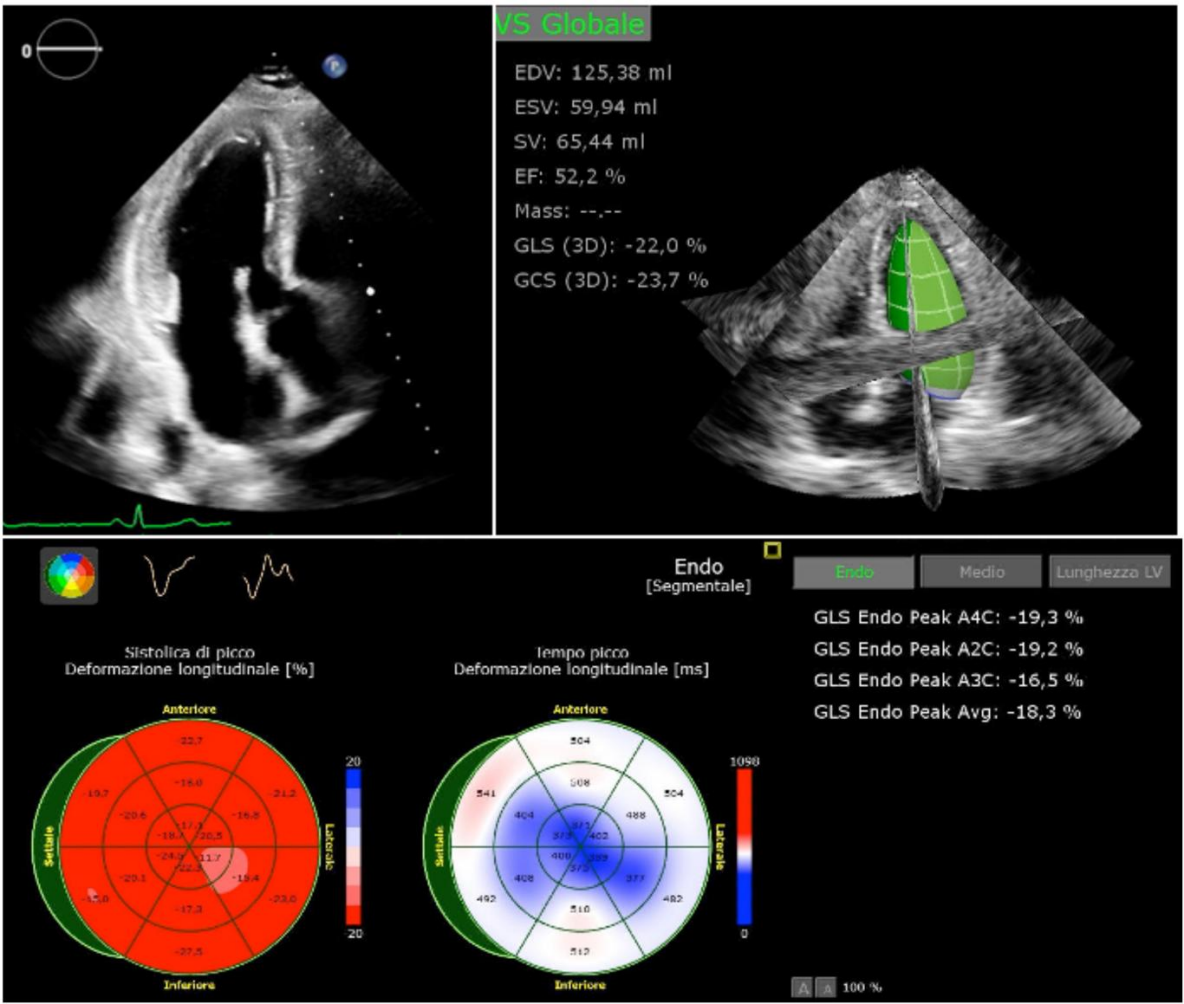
Transthoracic echocardiography. (*Top left panel*) Three-chamber view; (*Top right panel*) 3D echocardiographic ejection fraction and volumes; (*bottom panel*) percentage systolic peak longitudinal deformation index.
